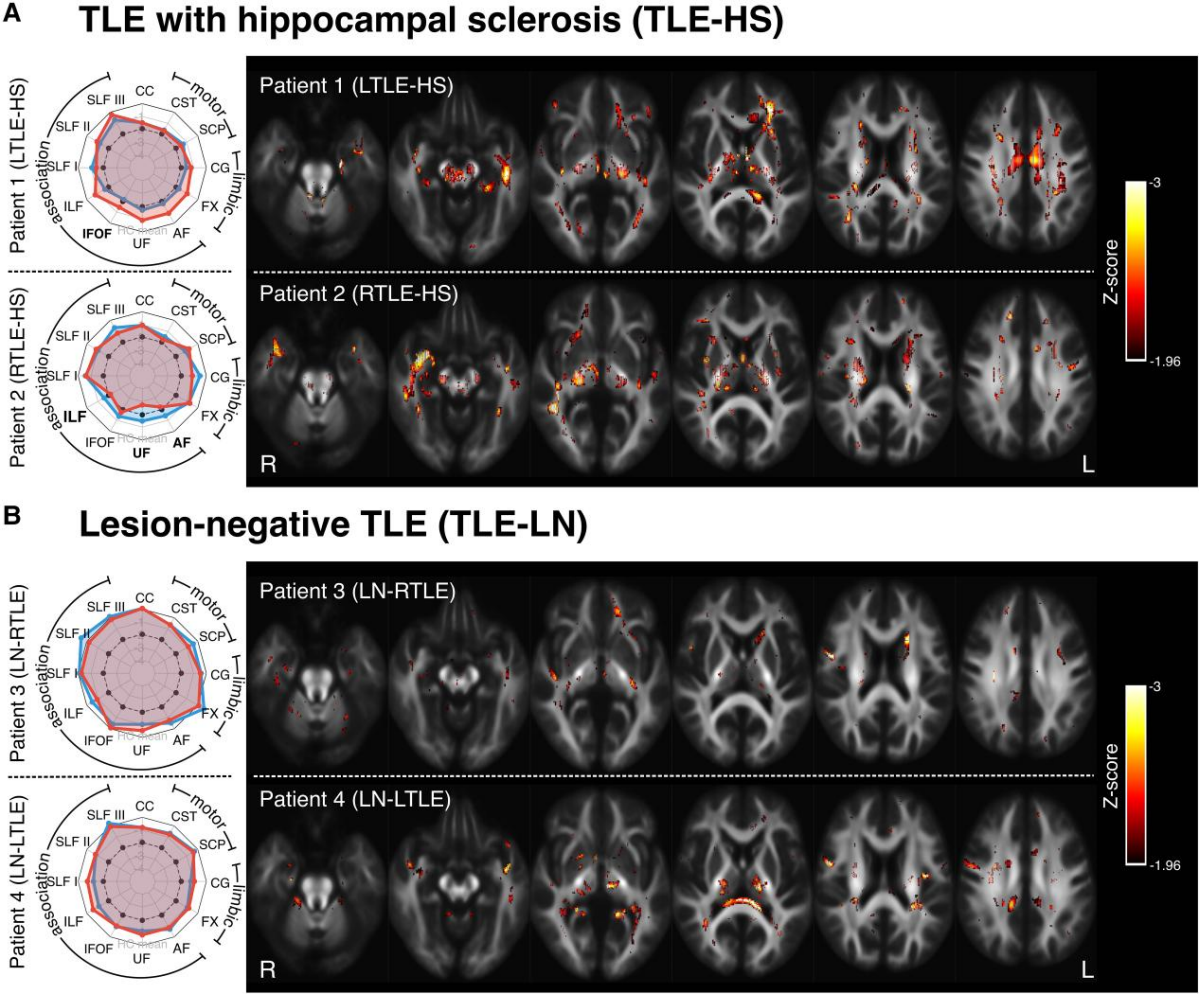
Tract- and fixel-level results for four patients with TLE. (A) Two patients with HS: Patient 1 is an individual who has left TLE with HS (L TLE-HS); Patient 2 is an individual with right TLE with HS (R TLE-HS). (B) Two patients with LN TLE: Patient 3 is an individual with MRI-negative or LN-RTLE; and Patient 4 is an individual with LN-LTLE. In all cases, spider plots on the left show Z-scores for FDC measures in each tract-of-interest (see Fig. 2 for interpretation). Fixel-level Z-score maps are shown on the right, with fixels exhibiting a Z-score of −1.96 or greater. Axial slices are placed at 10 mm increments. AF, arcuate fasciculus; CC, corpus callosum; CG, cingulum; CST, corticospinal tract; FX, fornix; IFOF, inferior fronto-occipital fasciculus; SCP, superior cerebellar peduncles; SLF, superior longitudinal fasciculus; UF, uncinate fasciculus.
